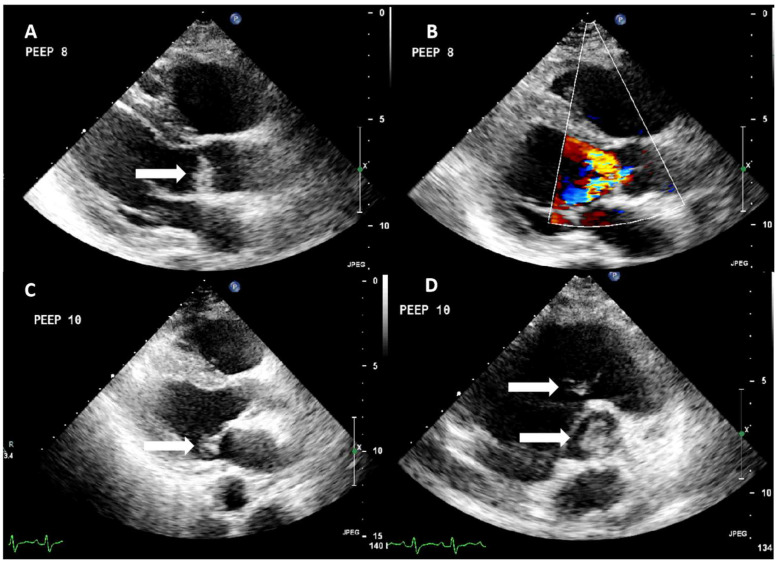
Echocardiographic findings presenting lesions in multiple valves. (A,B) Parasternal long-axis view showing a mass (vegetation) attached to the ventricular surface of the aortic valve (indicated by the white arrow). There is significant aortic regurgitation with two eccentric jets. (C) Parasternal long-axis view showing a mass attached to the atrial surface of mitral valve (white arrow). (D) Modified parasternal short-axis view focusing on the right chambers, showing a thin vegetation attached to the ventricular surface and the subvalvular apparatus of the tricuspid valve (white arrow). There is also vegetation attached to the aortic valve (white arrow).
